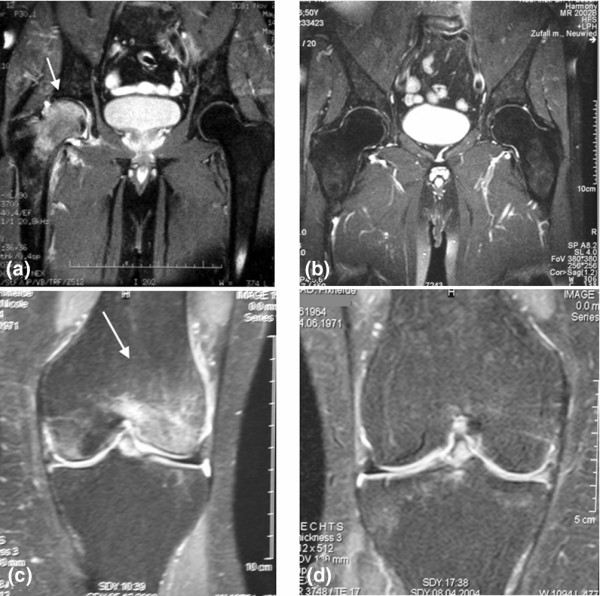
MRI scans (T2-weighted) of two different patients with bone marrow oedema (BME) (a, c) before and (b, d) six months after iloprost application. (a, b) The BME of a 50-year-old man with chronic alcohol abuse resolved completely after iloprost infusion. (c, d) A 32-year-old woman with painful BME of the medial condylus during immunosuppressive therapy after kidney transplantation was treated with iloprost and healed within six months.
